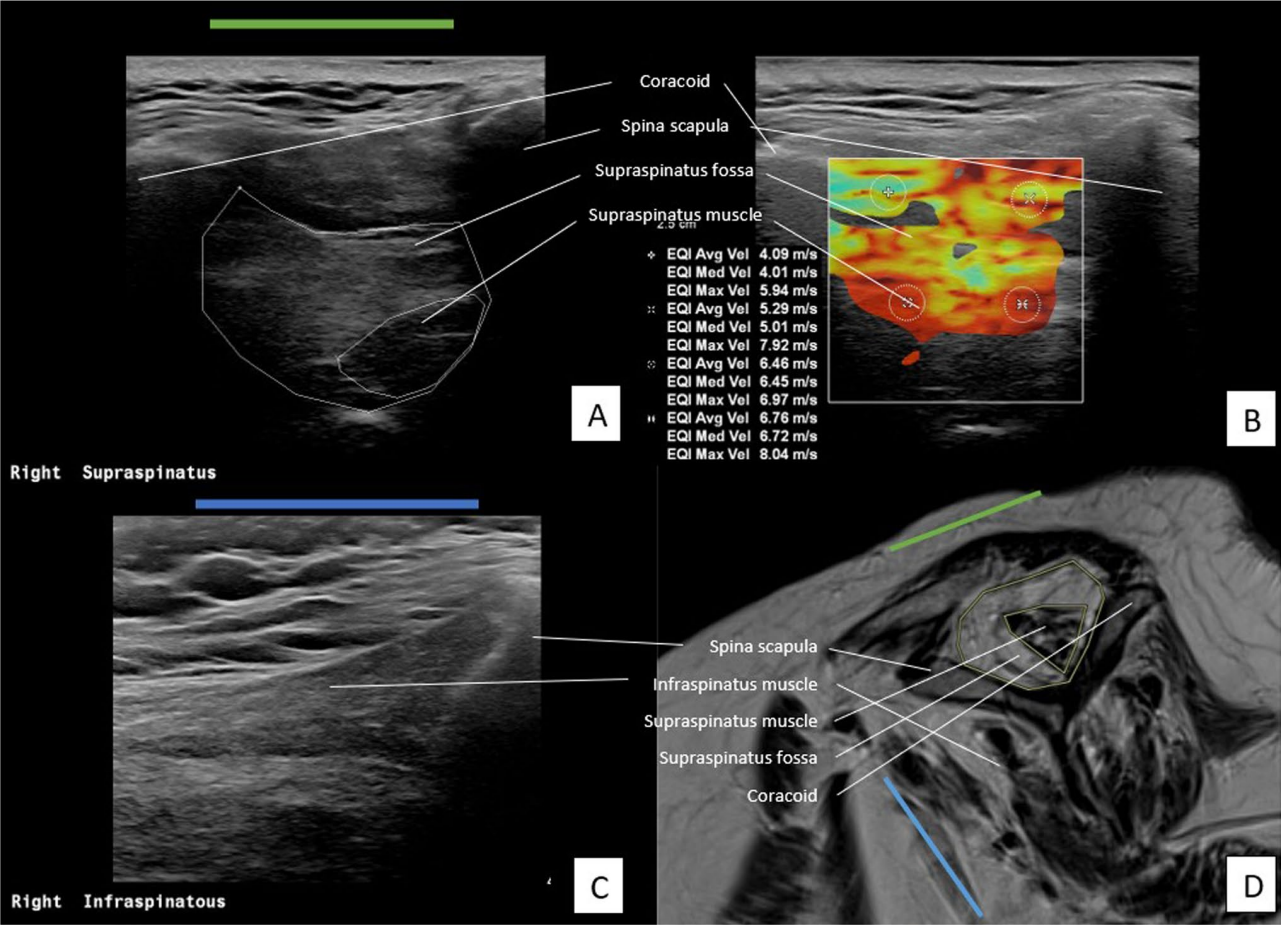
Assessment of SSP and ISP muscle on US and MRI of a patient with severe muscle atrophy and fatty infiltration of the SSP muscle. A US plane of SSP. B US plane of SSP with measurements of SWV in m/s in four quadrants. C US plane of ISP. D oblique-sagittal plane of MR image to assess SSP and ISP muscle
